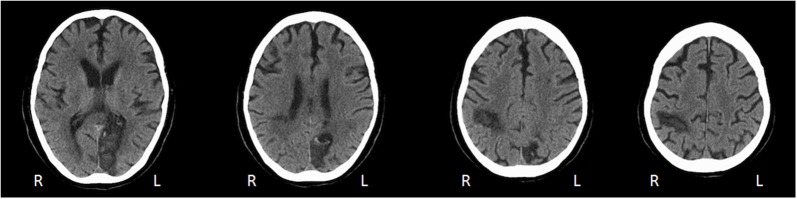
Axial CT scan performed 6 weeks after stroke reveals right parieto-occipital and left occipital lesions.
